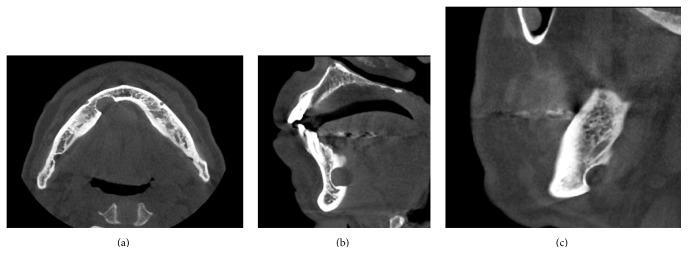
Cone-beam computed tomography (CBCT). (a) Axial image. Two defects of the cortex on the lingual plate of the mandible are apparent. (b) Sagittal image of the cavity in the premolar region, showing dense, radiopaque contents. (c) Sagittal image of the cavity at the angle of the mandible.
